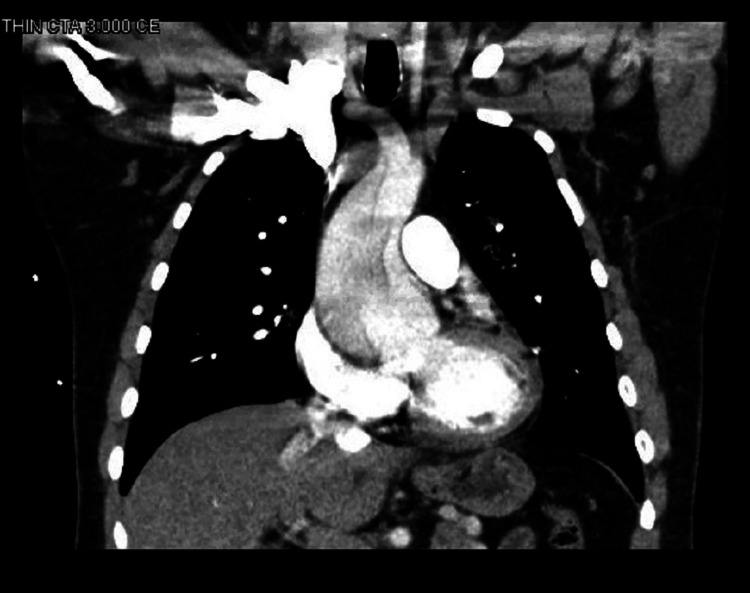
Coronal view of CT angiography of the chest showing aneurysmal dilatation of the aortic root approaching 6 cm with extensive DeBakey type 1 dissection
